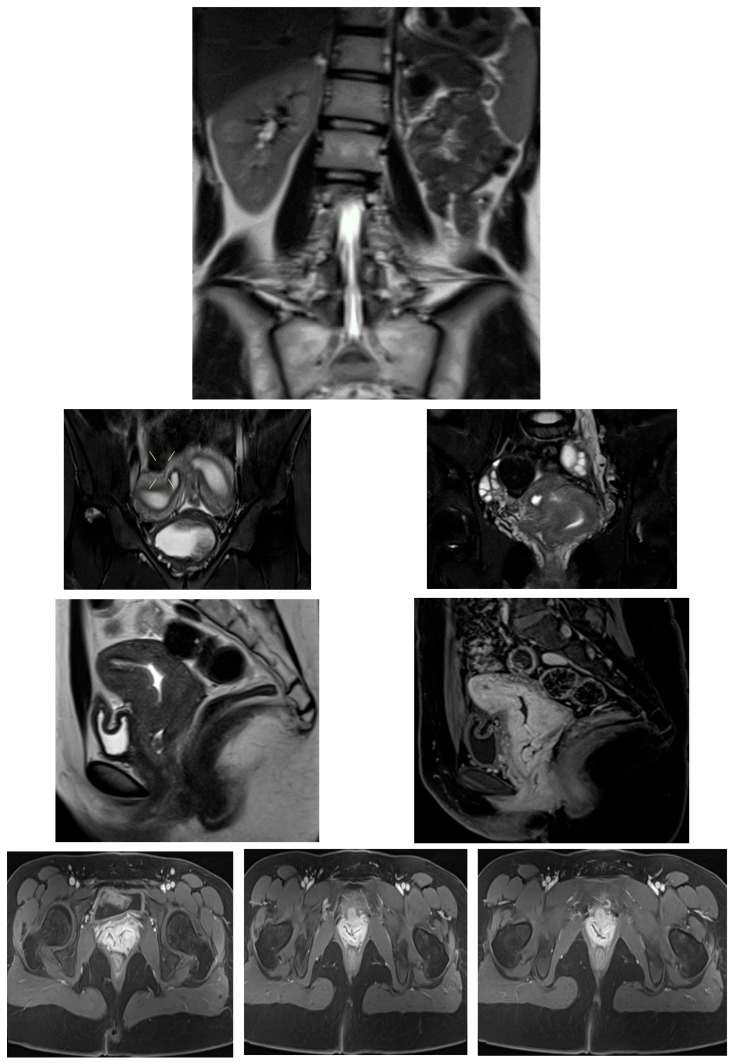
MRI pelvis in Case 2 demonstrating uterine didelphys with left distal hemivaginal obstruction and a large blood-filled elongated vagina/hematocolpos forming a pelvi-abdominal cystic mass. Absence of the left kidney is noted, consistent with OHVIRA syndrome.
